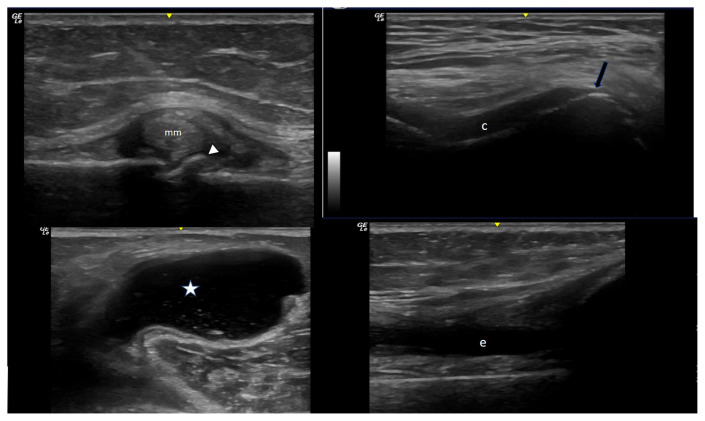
Sonographic findings in knees with OA include osteophytes (white arrowheads), suprapatellar effusion (e), protrusion of the medial meniscus (mm) with displacement of the medial collateral ligament, a Baker’s cyst (star), and decreased cartilage (c) thickness (arrow).
